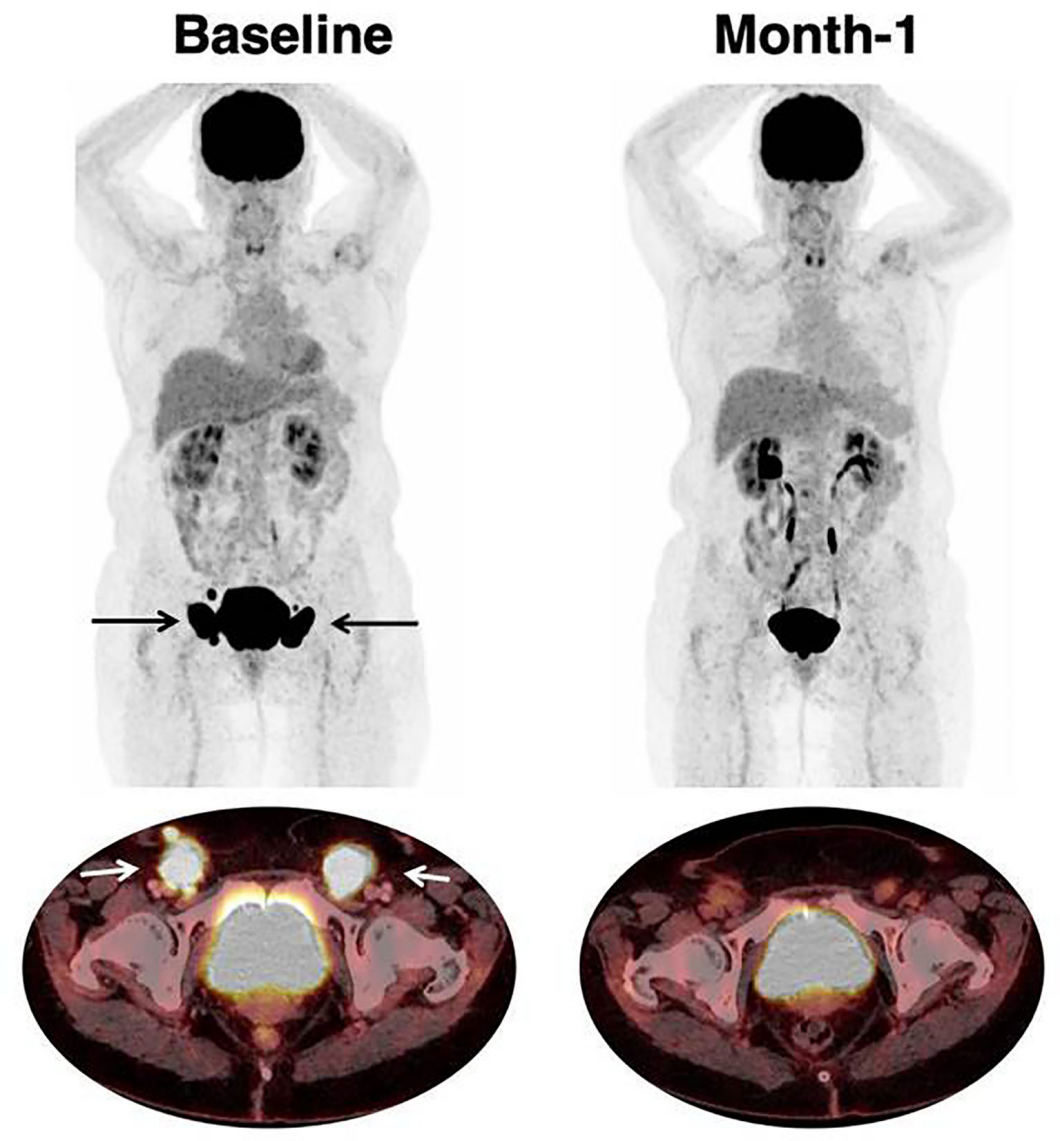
Response to CAR T-cell therapy. 66 year-old patient with past medical history of follicular lymphoma. The patient relapsed with DLBCL, treated with two lines of prior chemotherapy. Baseline imaging showed a low tumor volume (TMTV was 47 mm^3^) which is typically associated with favorable outcome and response to CAR T-cell therapy. Inguinal lymphadenopathies are indicated with black (on Maximum Intensity Projection) and white arrows (on axial fusion image). Follow-up imaging showed a partial response on CT-scan with residual disease. [18F]-FDG PET reclassified this patient as a complete metabolic response which persisted at month-6.
